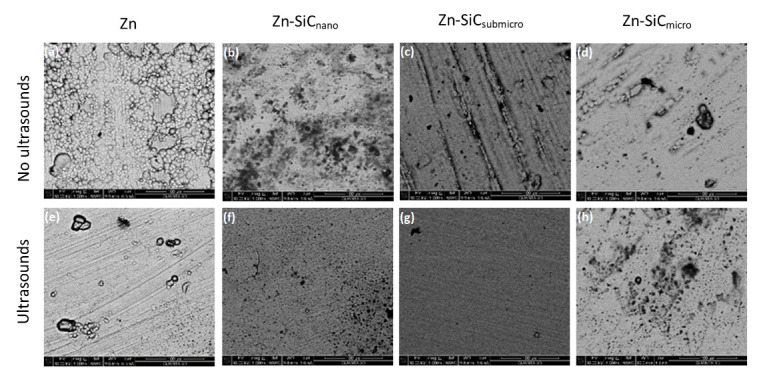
SEM images of Zn and Zn-SiC coatings deposited at 1 A/dm^2^ without (a–d) and in the presence of ultrasound (e–h) (mag. 2.5k).
